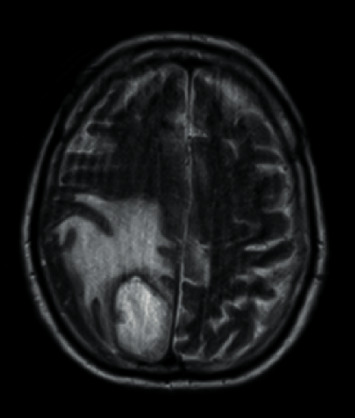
CE-CT in the latter hospital.
